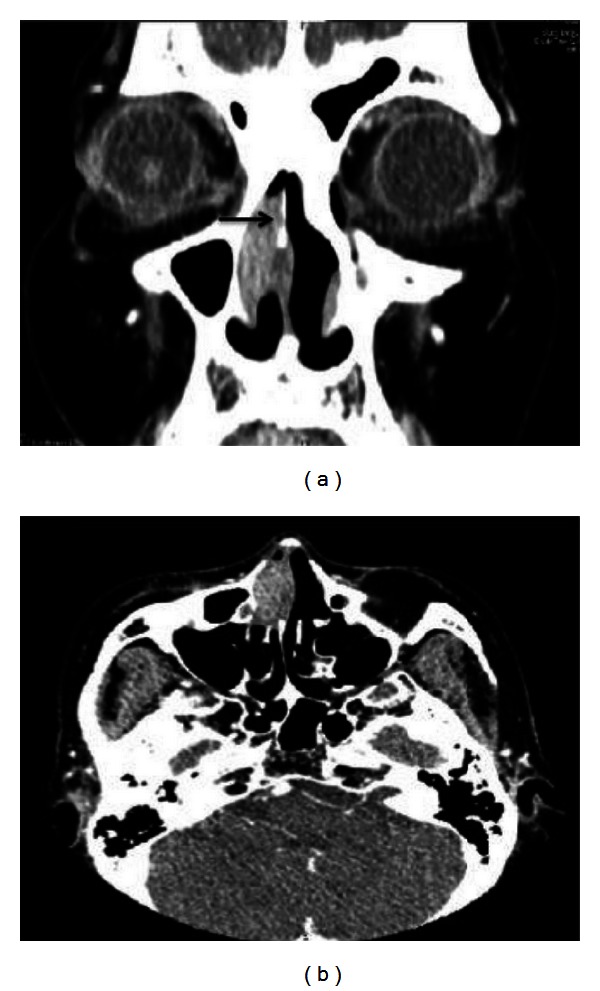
Coronal (a) and axial (b) images of paranasal sinus computed tomography. The mass is heterogeneously iso-intense between the nasal septum and lateral nasal wall, with erosion of septal bone (arrow).
